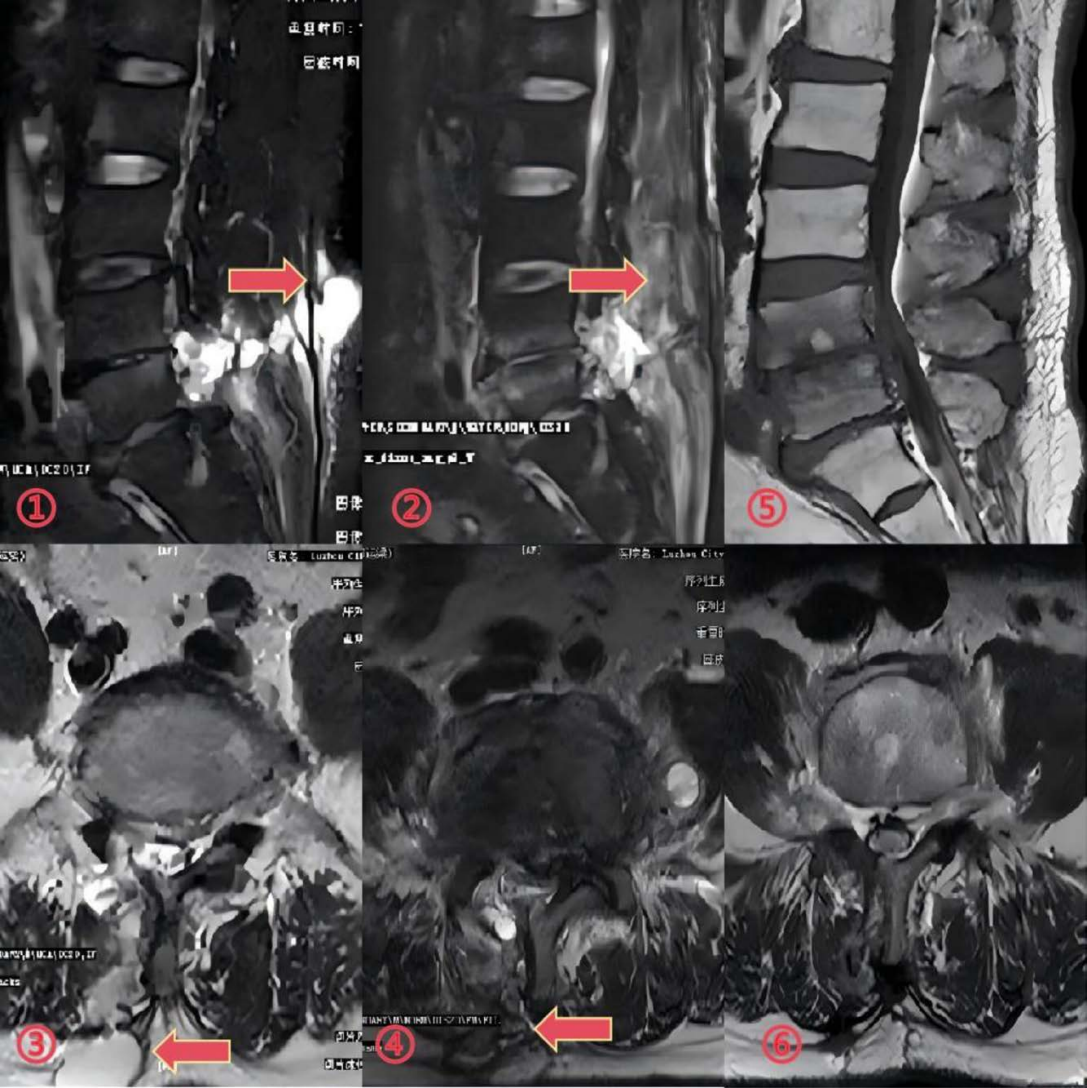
①③: Preoperative MRI examinations were performed prior to lumbar drainage. ②④: One week after lumbar drainage, follow-up MRI examinations confirmed resolution of the cerebrospinal fluid (CSF) leak. ⑤⑥: Three months postoperatively, MRI examinations were repeated to assess long-term outcomes. MRI = magnetic resonance imaging.
